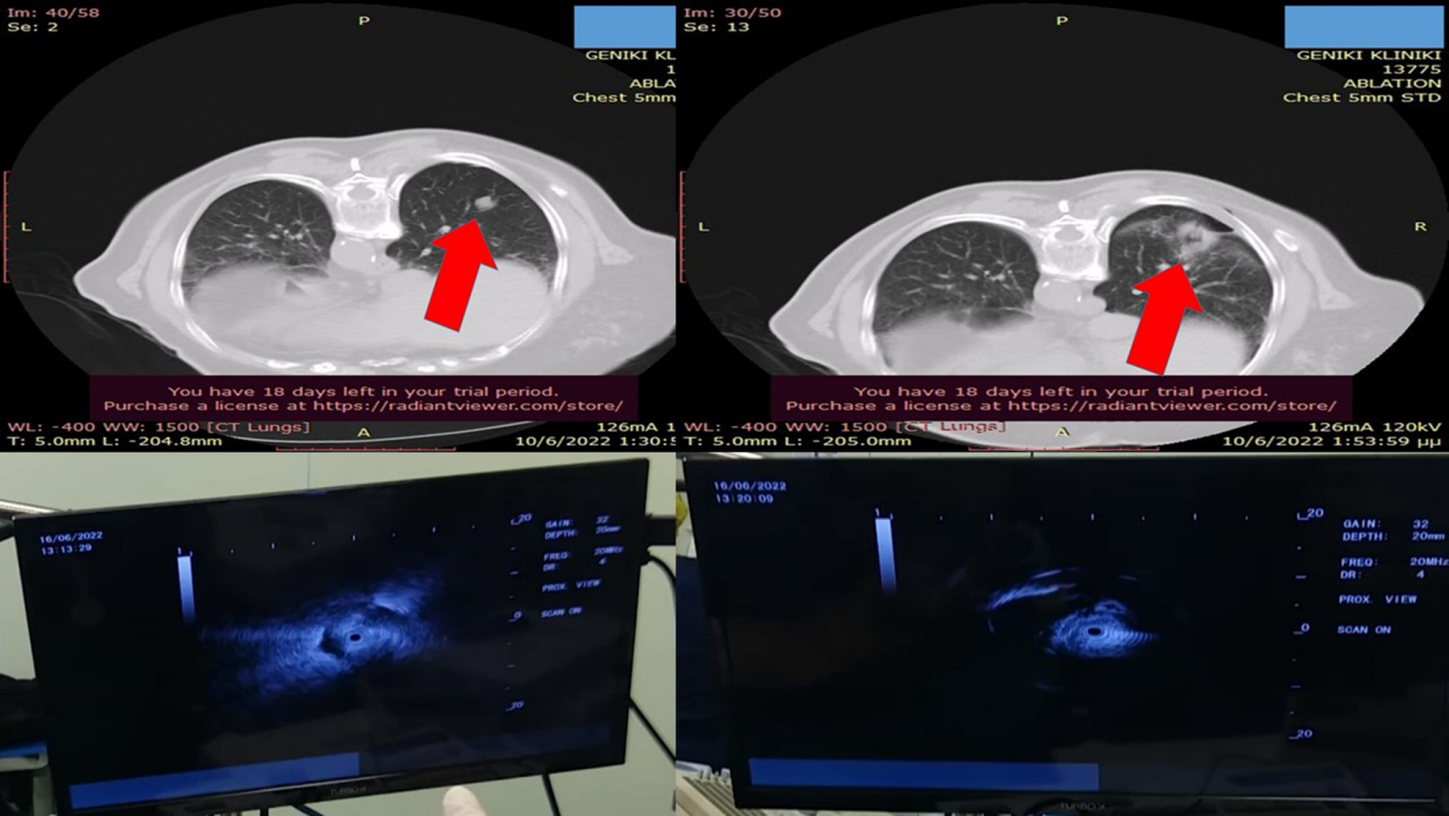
Left upper-row red arrow indicates the lesion before ablation, the left lower row demonstrates the lesion sign with radial-EBUS, the right upper-row red arrow indicates the area after two sessions of thermal effect with radiofrequency ablation, and the right lower row demonstrates the lesion sign after the ablation. EBUS, endobronchial ultrasound.
